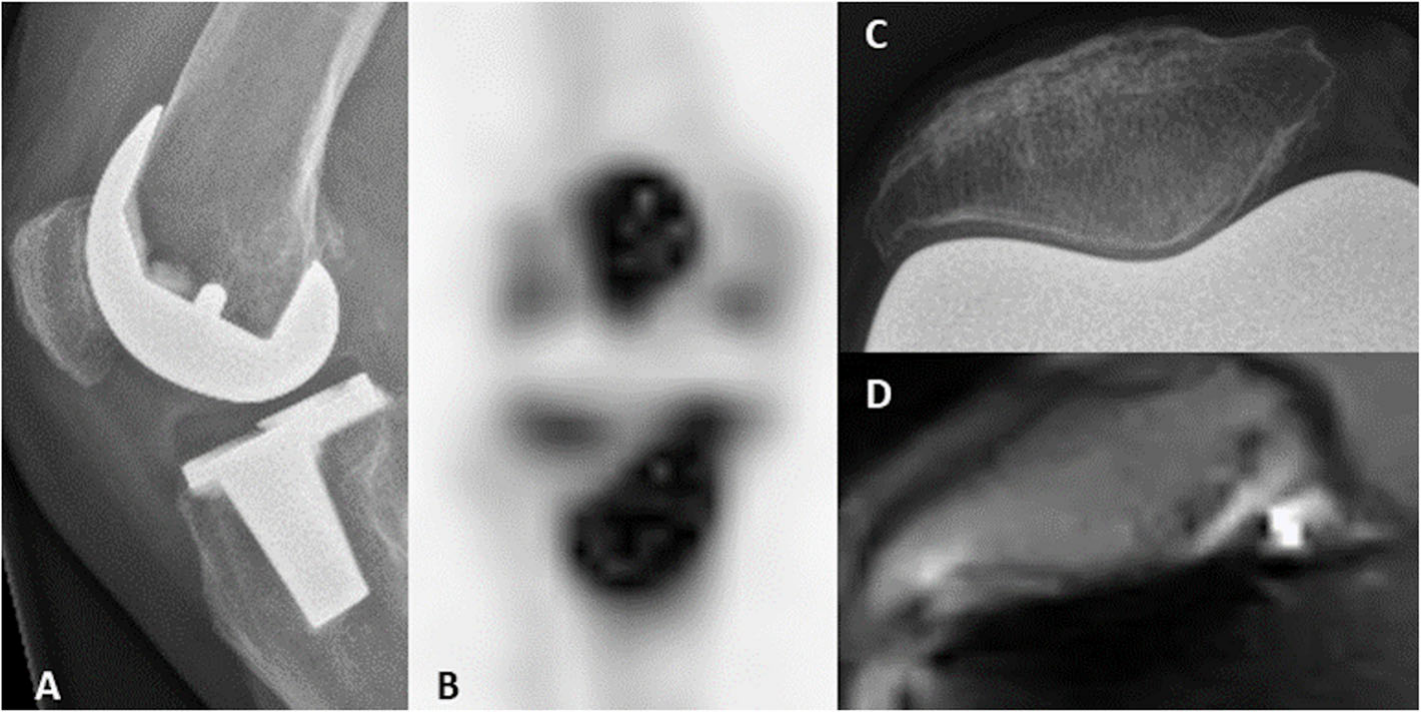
Patellofemoral arthrosis. The conventional radiograph (a) of patient 8, together with the bone scintigraphy (b), the additional patellofemoral radiograph (c) and the low-field MRI (d). Except for the conventional radiographs (a), all other images b-d) show patellofemoral arthrosis
